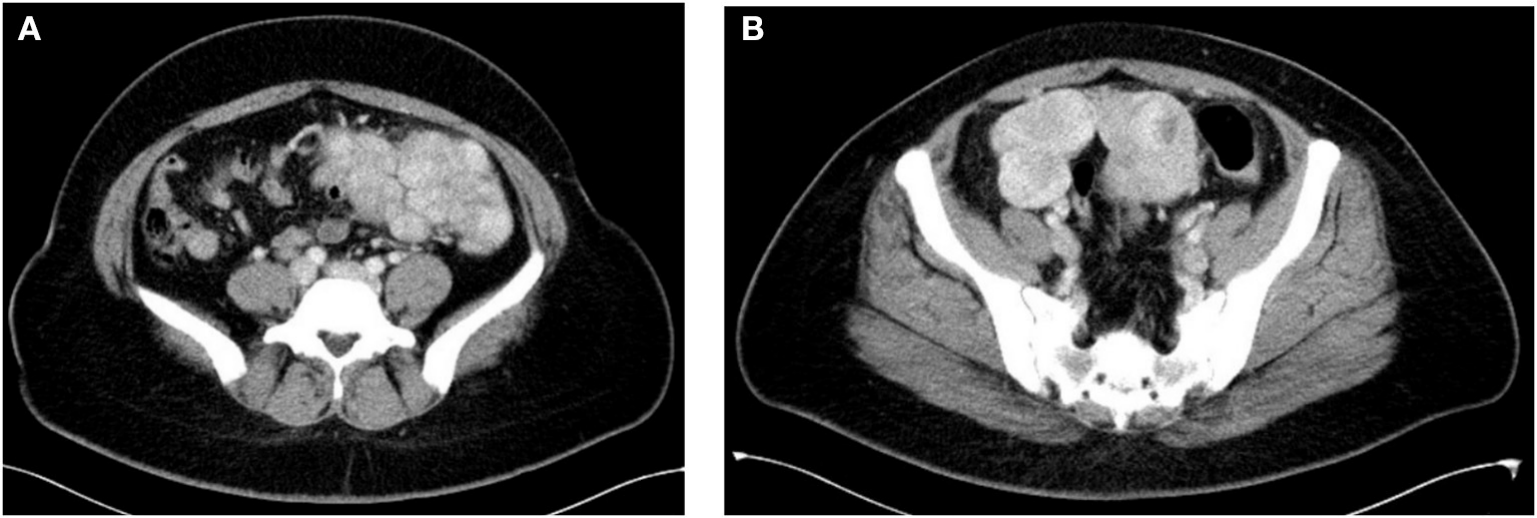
(A,B) Axial contrast enhanced CT showed multiple soft tissue lesions in mesentery with necrosis in some lesions (Case 4).
